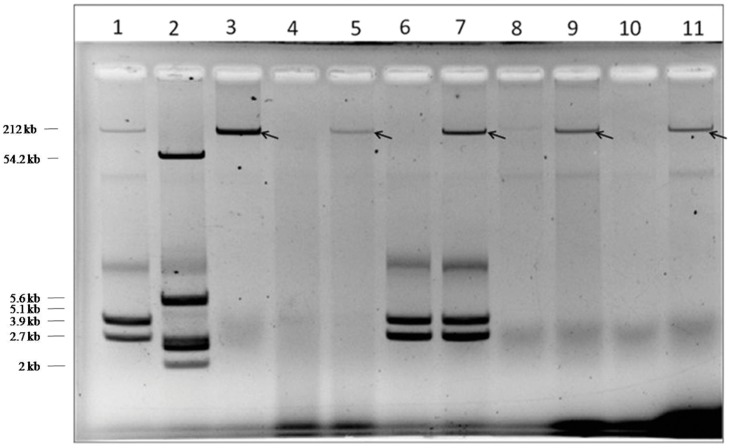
Plasmid profile of donor, recipient, and transconjugants. Lanes 1 and 2, *Shigella flexneri* YSH 6000 and *Escherichia coli* K12 V517 were used as reference standards, respectively; Lane 3, BCH 2406: *Salmonella* Senftenberg (donor); Lane 4, IDH 5313: *Vibrio cholerae* O1 Ogawa (recipient); Lane 5, CT-*V. cholerae* (transconjugant); Lane 6, IDH 3077: *S. flexneri* 2a (recipient); Lane 7, CT-*S. flexneri* (transconjugant); Lane 8, XL1-Blue, *E. coli* (recipient); Lane 9, CT-*E. coli* XL1-Blue (transconjugant); Lane 10, *E. coli* J53 (recipient); Lane 11, CT*-E. coli* J53 (transconjugant).
